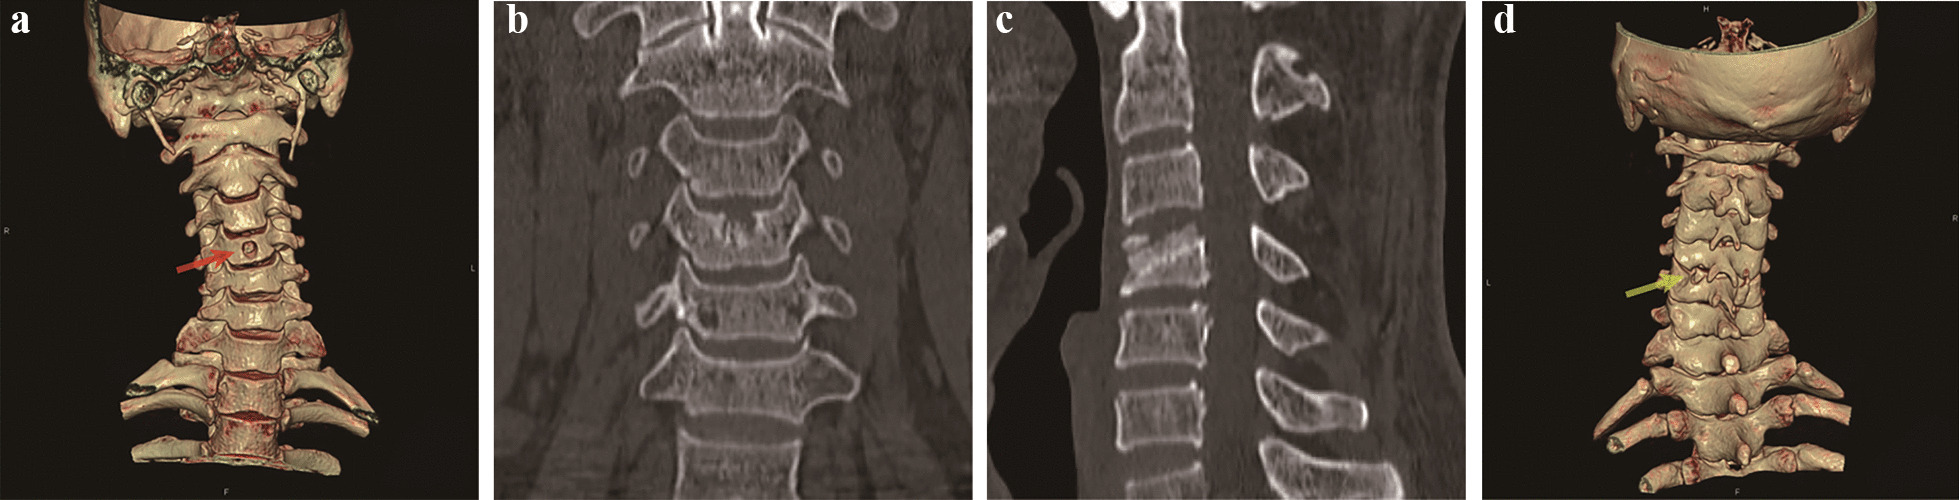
CT images of the patient 1 week after surgery. a CT 3D reconstruction showing the location of the vertebral bone channel entrance (red arrow). b Coronal view showing the C4 vertebral body bone channel and the internal autogenous bone graft. c Sagittal view showing the C4 vertebral bone channel and the internal autologous bone graft. d CT 3D reconstruction showing the entrance to the C5 lamina bone window (yellow arrow)
